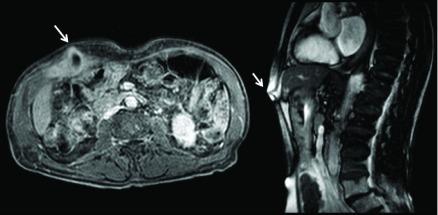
Hepatobiliary MRI. The white arrows show the fistula. From left to right: coronal and sagittal fistula view.
